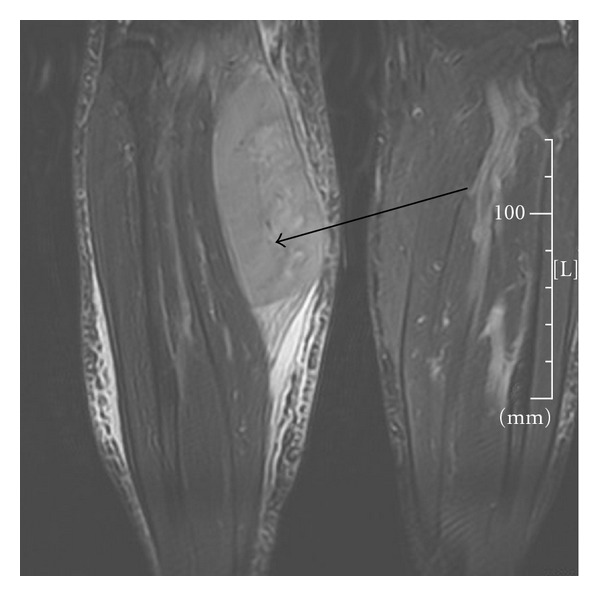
MRI of the lower extremities with right leg mass involving the medial gastrocnemius muscle.
